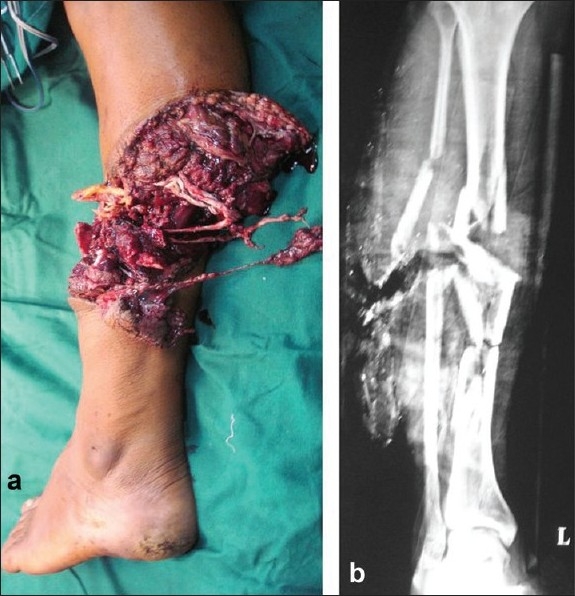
(a) Clinical photograph of leg with severe crushing of soft tissues with absence of a vascular injury. (b) X-ray of left leg bones (AP view) was showing comminuted fracture tibia with bone loss. MESS has poor senstivity for amputation. Attempted salvage of this leg wound have led to prolonged surgeries and probably a secondary amputation. In contrast, Ganga Hospital Score was 17 indicating the need for amputation. In IIIB injuries, Ganga Hospital Score was more sensitive than a MESS in predicting amputation
